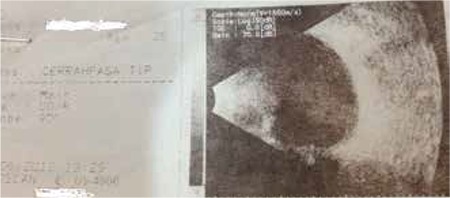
Preoperative B-scan ultrasonography image
